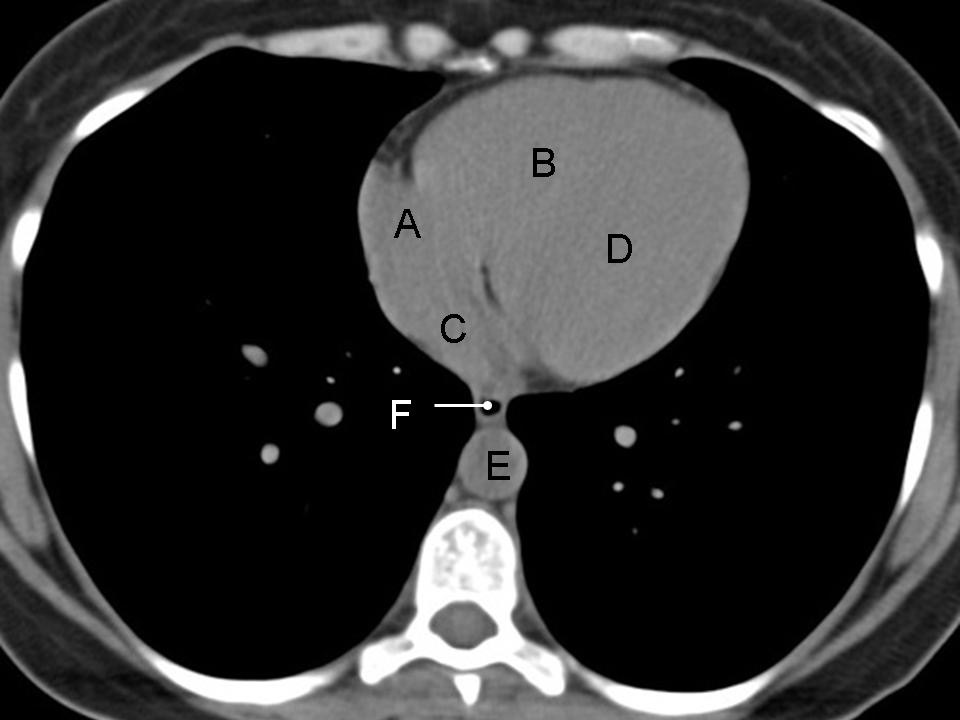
Non-contrast CT of the chest of a 34 years old male shows normal appearance of right atrium (A), right ventricle (B), left atrium (C), left ventricle (D), descending thoracic aorta (E) and oesophagus (F). Note that outlines of individual cardiac chambers and inter-ventricular septum are not clearly delineated on the non-contrast image. Compare the appearance of the oesophagus with that in the Figure 1 to appreciate the difference between its collapsed and air-filled segment
